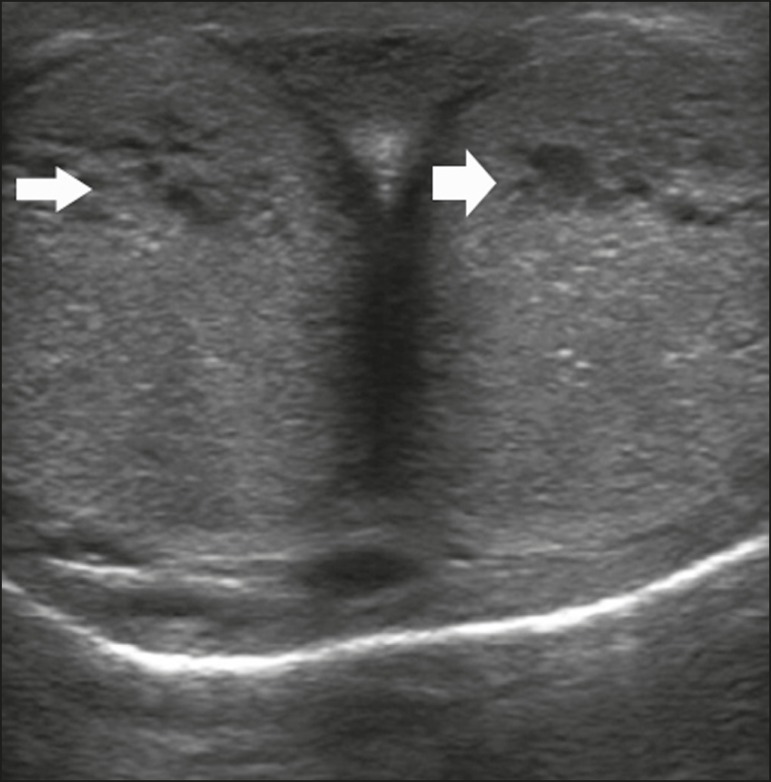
Transverse ultrasound image, ventral view of the penis. Image obtained
